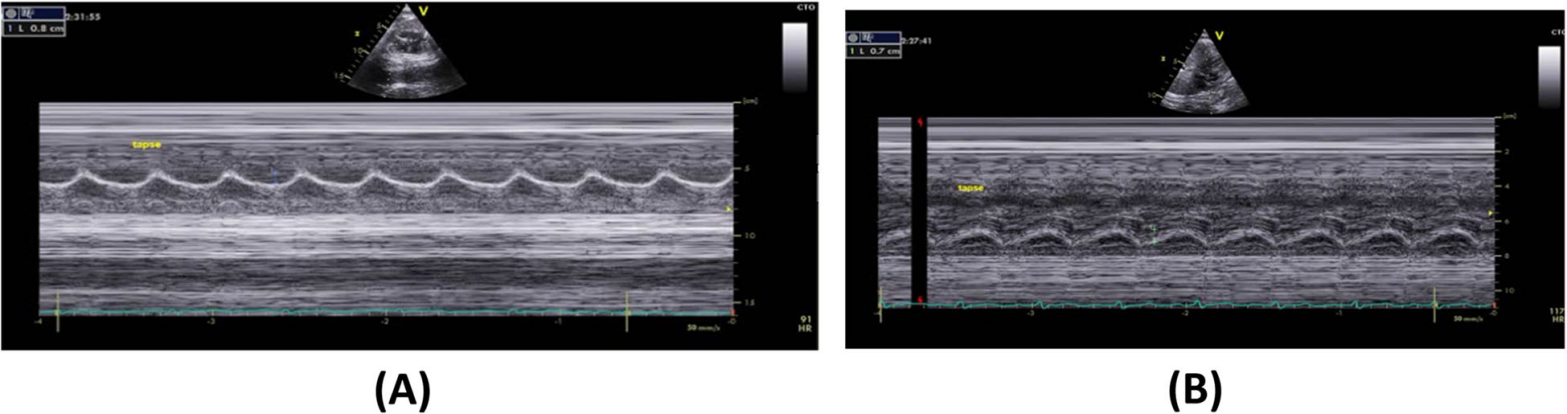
Tissue Doppler septal annular plane systolic excursion (SAPSE) in the fetus of a diabetic mother and b obese mother
